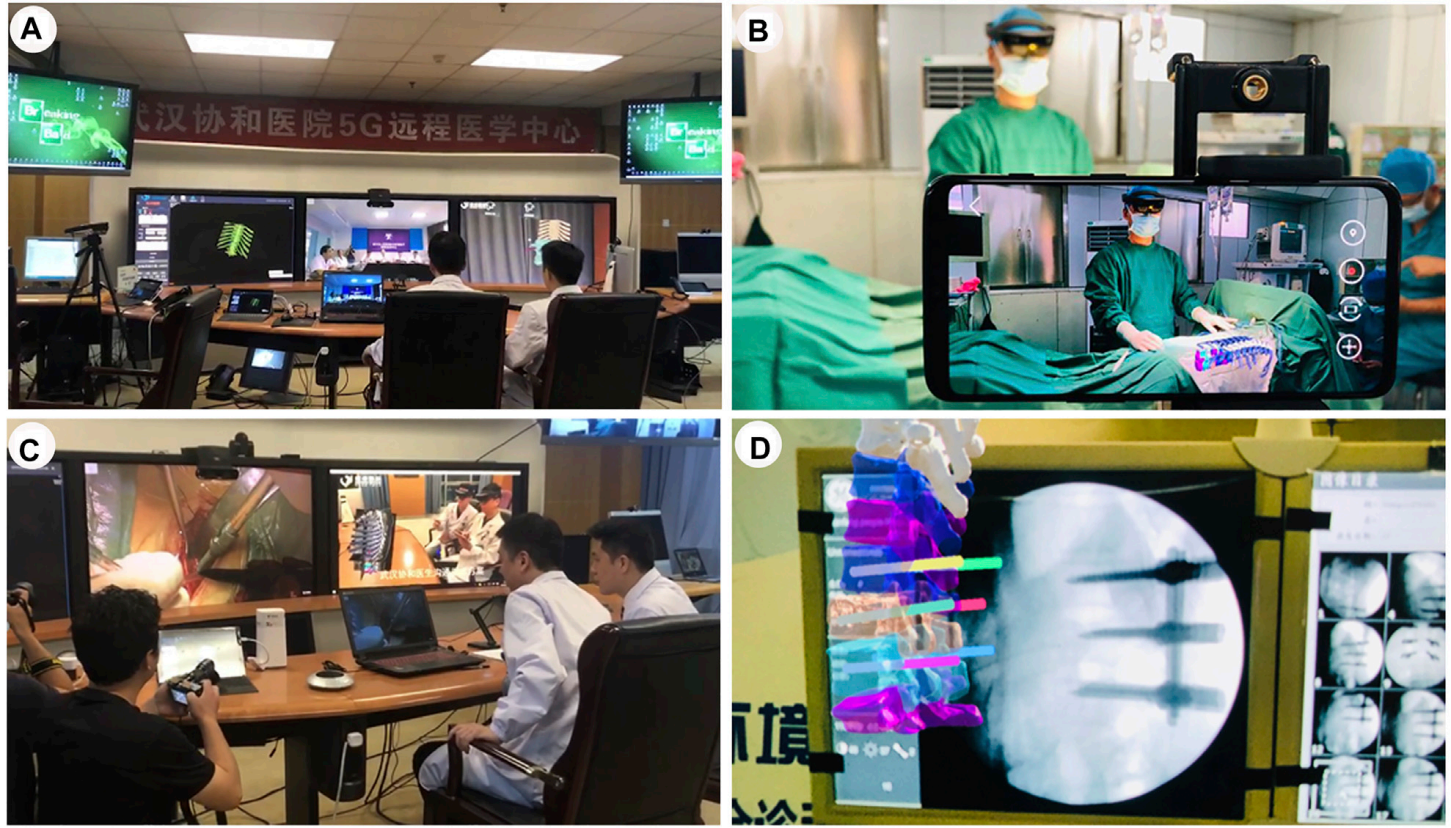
A cloud-based 5G-network mixed-reality platform for telesurgery. (A). The patient’s CT data was imported into Mimics, and a 3D model of the fractured spine was rapidly reconstructed. (B). During the surgical procedure, doctors from different places shared MR holograms in real time through a 5G network. (C). Real-time intraoperative guidance by remote experts. (D). Pedicle screws inserted with high precision into the T12 fracture.
